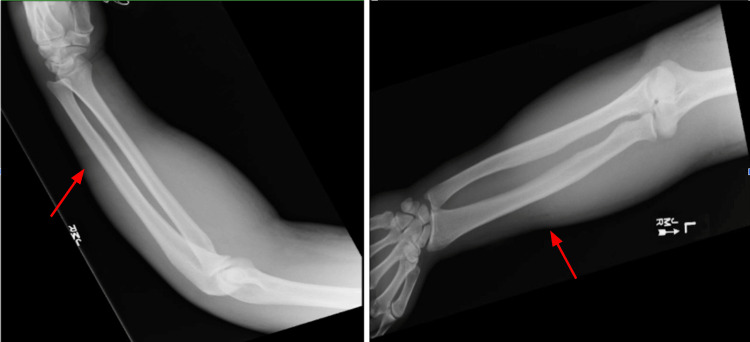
Plain radiographs of the left forearm Radiographs demonstrating subcutaneous emphysema (arrow), a key radiographic clue supporting necrotizing fasciitis.
